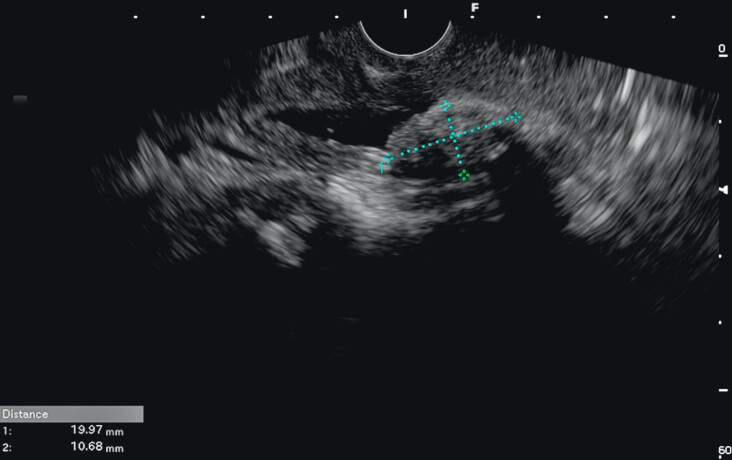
Endoscopic ultrasound showing dilation of the CBD with multiple stones, the largest measuring 19.97 mm × 10.68 mm. CBD, common bile duct.
